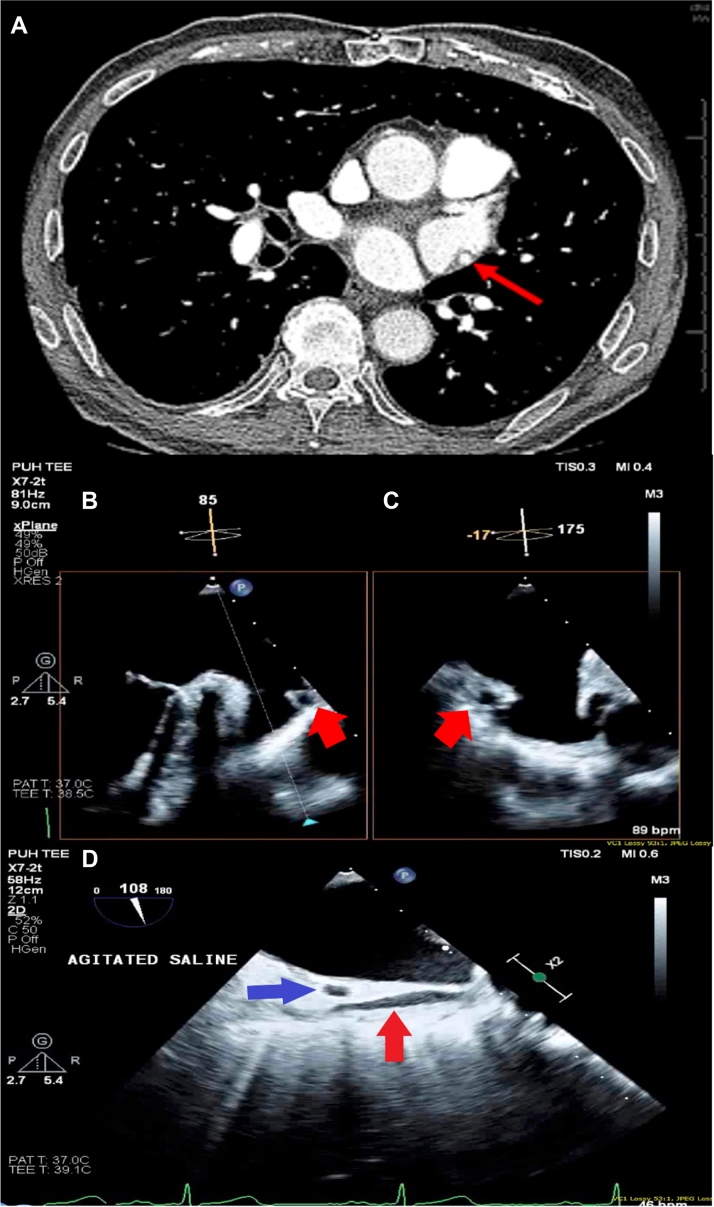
Images of Bypass Graft in 2 Imaging Modalities (A) Prior computed tomography angiography showing saphenous vein graft adjacent to left atrial appendage (LAA). Axial slice with red arrow pointing to bypass graft. (B to D) Left atrial appendage LAA views on transesophageal echocardiography at 85°, –17°, and 108°. LAA, anomalous vessels seen in 3 transducer angles along with cross-section of left circumflex artery. Blue arrow = left circumflex artery. Red arrow = anomalous vessel.
